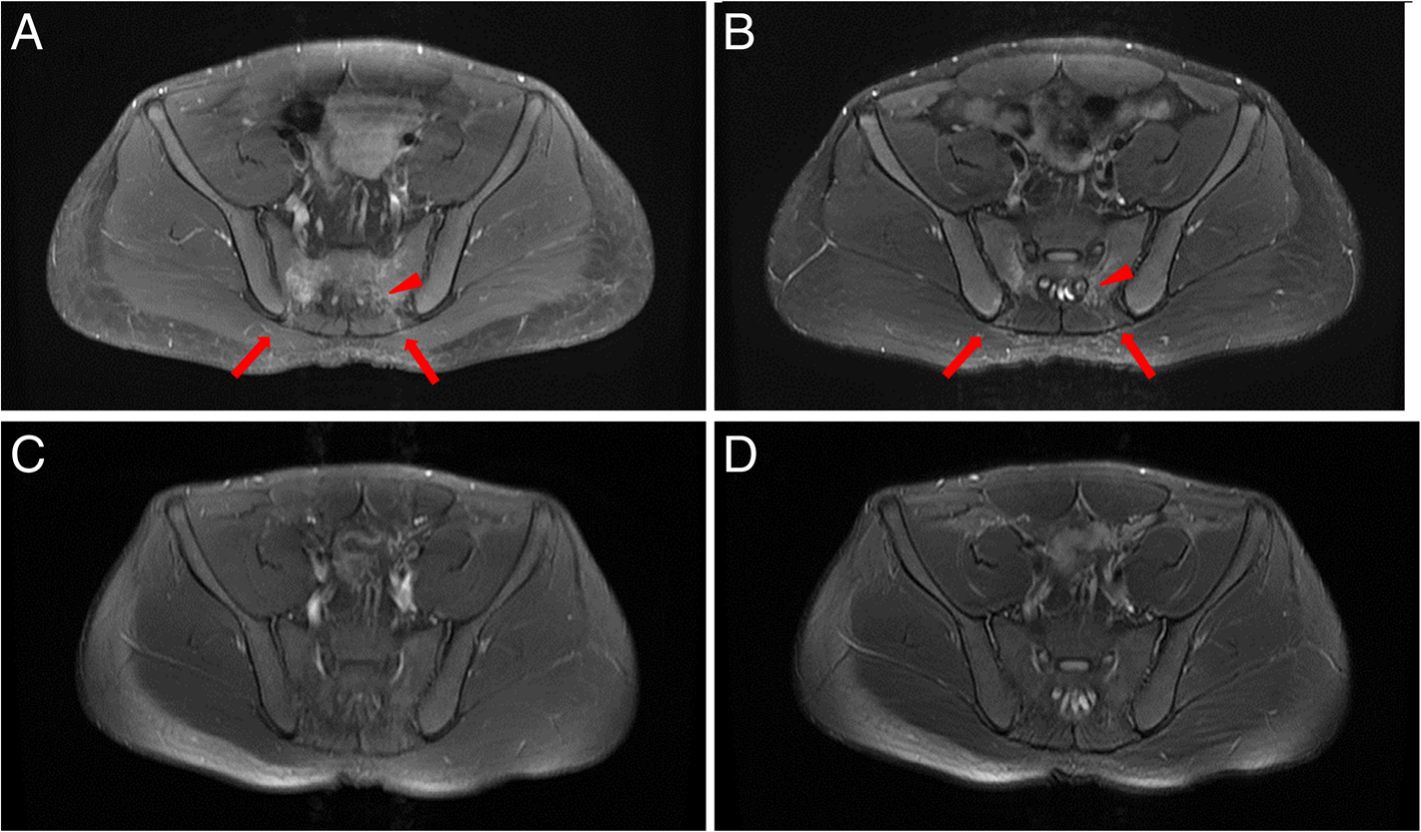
Pelvic magnetic resonance image (MRI) revealed left S2 nerve root injury. Initial T1 weighted image (a) and T2 weighted image (b) show enhancement around the S2 neural foramen (arrow head) and patchy edematous change with enhancement in the sacrum, retrosacral muscles, and subcutaneous layer around trajectory of the needle (Arrows). T1 weighted image (c) and T2 weighted image (d) of the follow up pelvic magnetic resonance image (MRI) 3 months after the onset. Little residual enhancement was present around the left S2 nerve root. However, edematous change and enhancement of the muscle and subcutaneous layer were not observed
